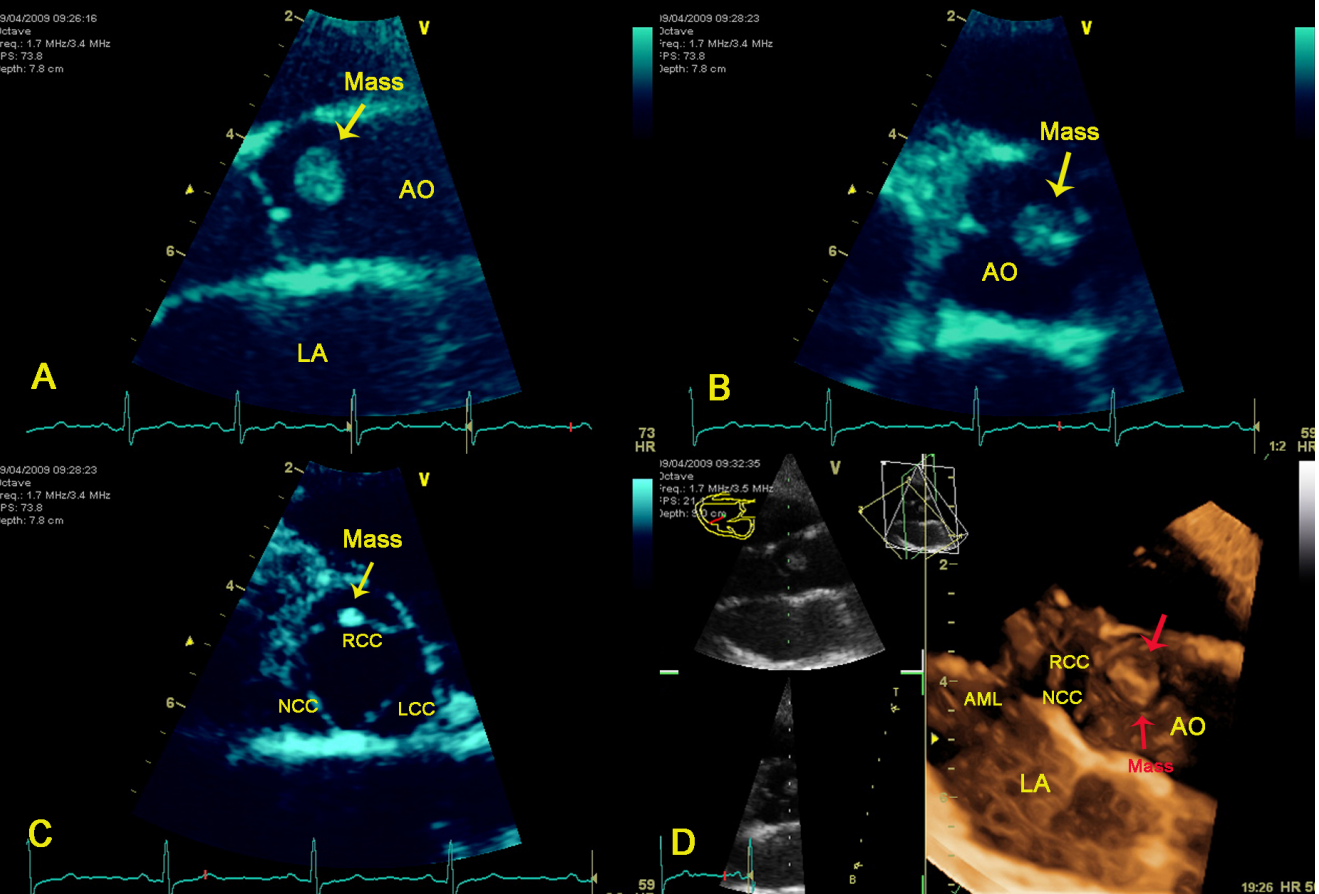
A. Long axis view of the aortic valve. Conventional 2D-echocardiography showing a large mass originating from the aortic surface of the valve. B. Short axis view of the aortic valve demonstrating in diastole a large papillary fibroelastoma of the right coronary cusp. C. Short axis view of the aortic valve demonstrating the right coronary cusp papillary fibroelastoma in systole. D. 3D-echocardiography depicting the origin of the tumour from the right coronary cusp. AO = Aorta, LA = Left atrial, RCC = Aortic right coronary cusp, NCC = Aortic non coronary cusp, LCC = Aortic left coronary cusp, AML = Anterior mitral leaflet.
